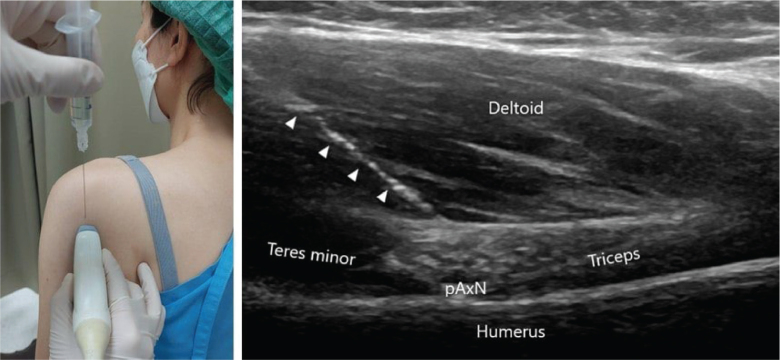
Ultrasound-guided dextrose PIT at quadrangular space (pAxN) white arrowhead, needle; pAxN, proximal part of the axillary nerve.
